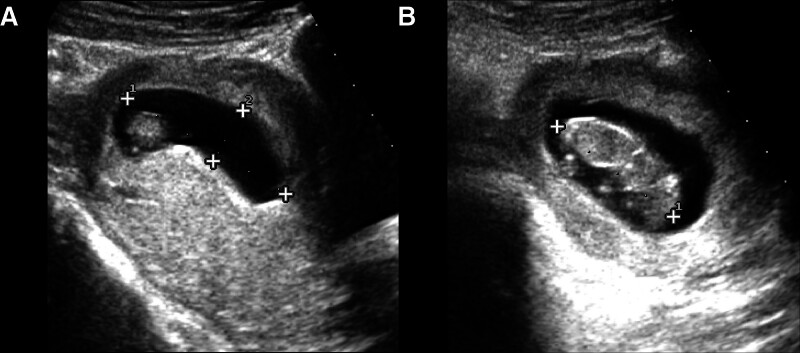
TAS results on postoperative day 8 (day 74 of amenorrhea). (A) Showing IP with a gestational sac size of 52 × 16 × 44 mm. (B) Embryonic pole size of 41 mm in length. IP = intrauterine pregnancy, TAS = transabdominal sonography.
